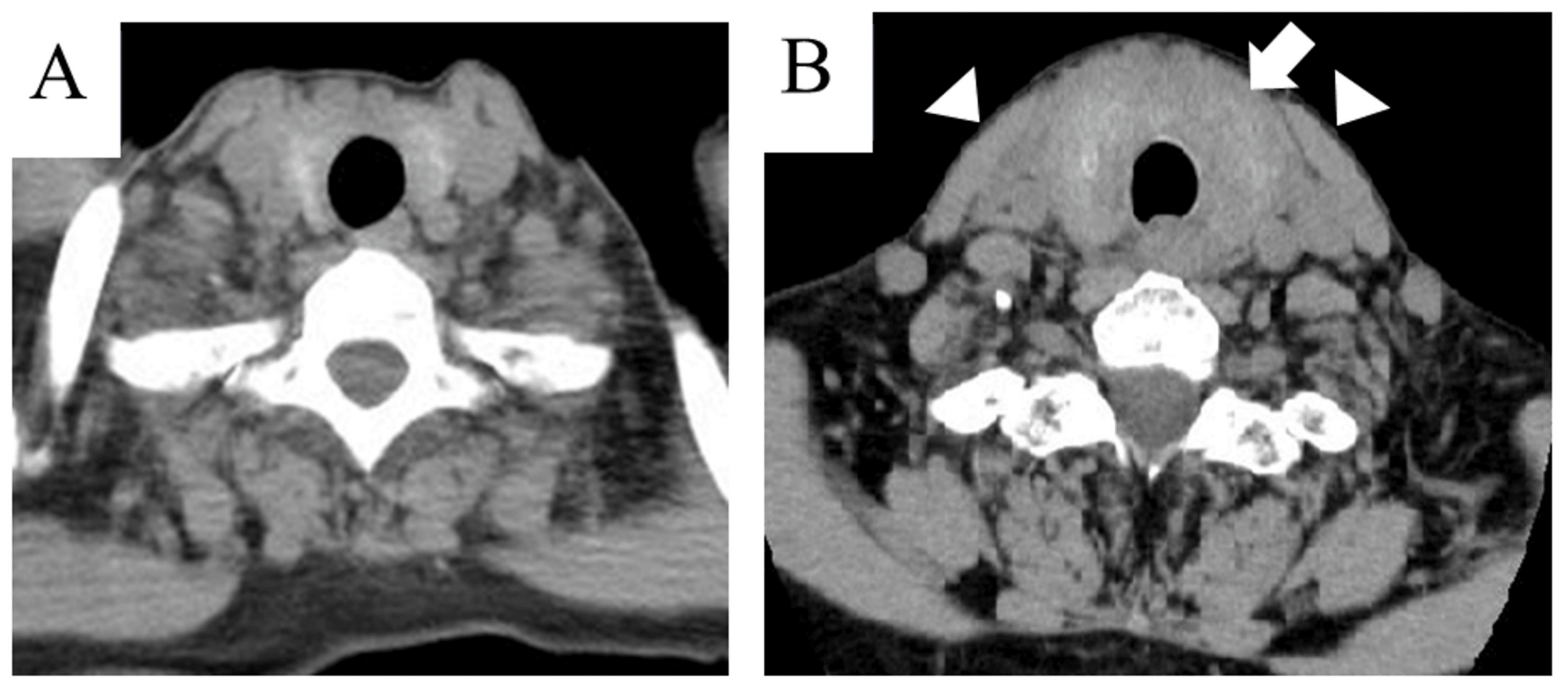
Computed tomography scan. ATTS before (A) and after (B) ATTS in patient #27. The white arrow and arrowheads depict the swollen thyroid and the swollen per-thyroid fat tissues, respectively. ATTS: acute transient thyroid swelling
